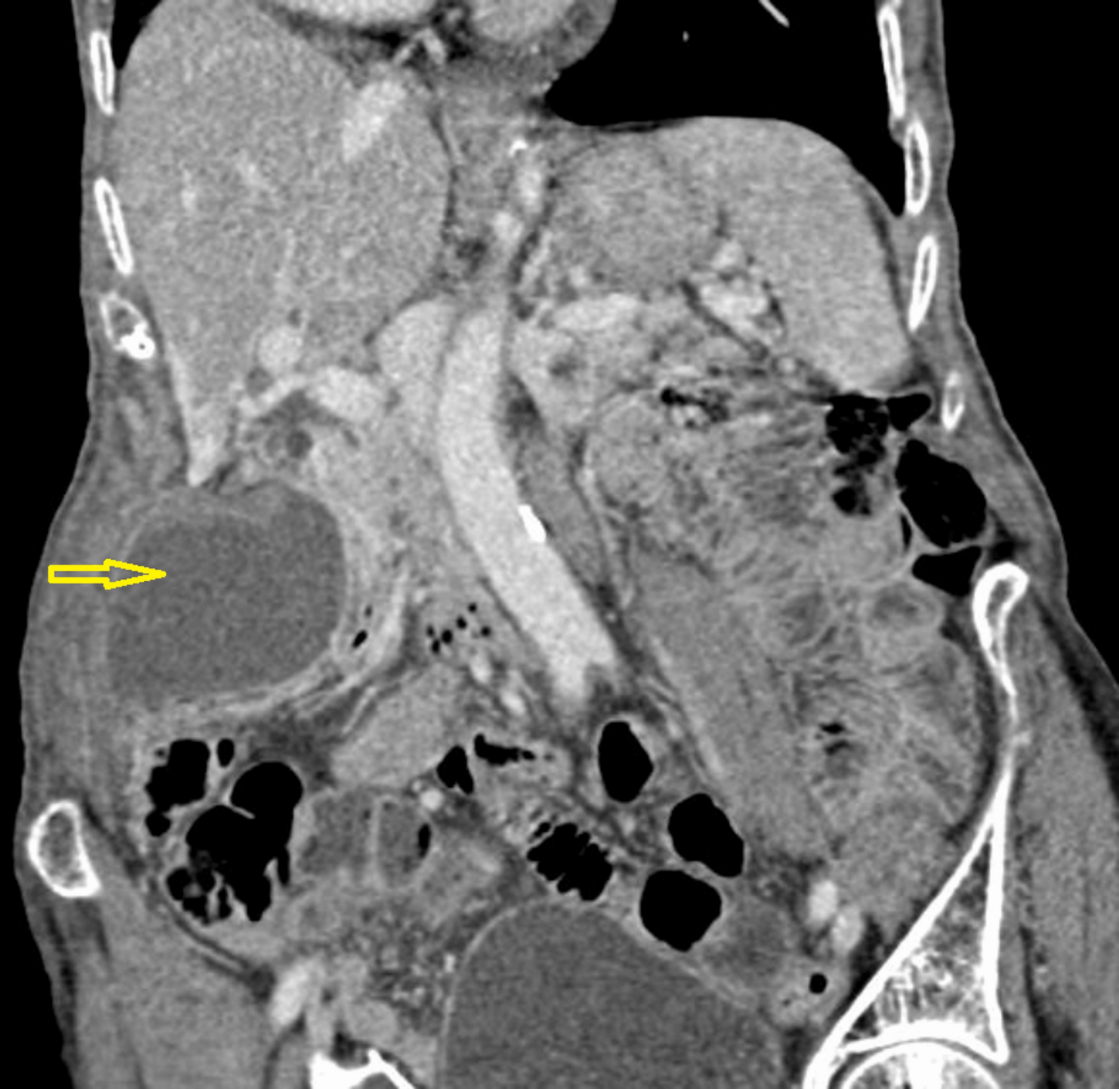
Coronal CT scan showing abnormal arrangement of the distended gallbladder Yellow arrow: distended gallbladder; CT: computed tomography
